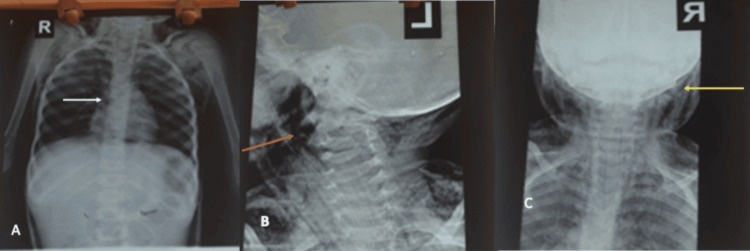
(A) Chest X-ray showing perihilar opacities in the chest. (B, C) X-ray of the neck displaying extensive radiolucency within the subcutaneous tissue (red arrow) and soft tissue swelling (yellow arrow)
